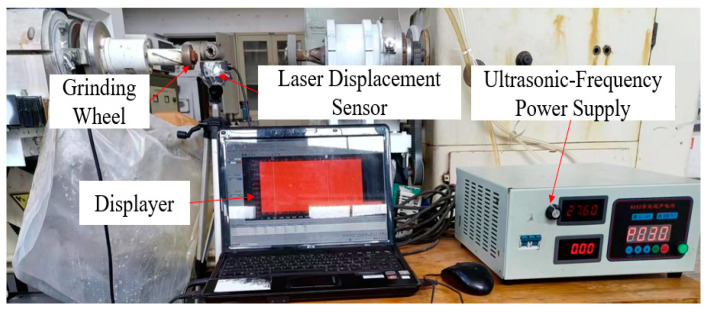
On-site ultrasonic amplitude measurement.
